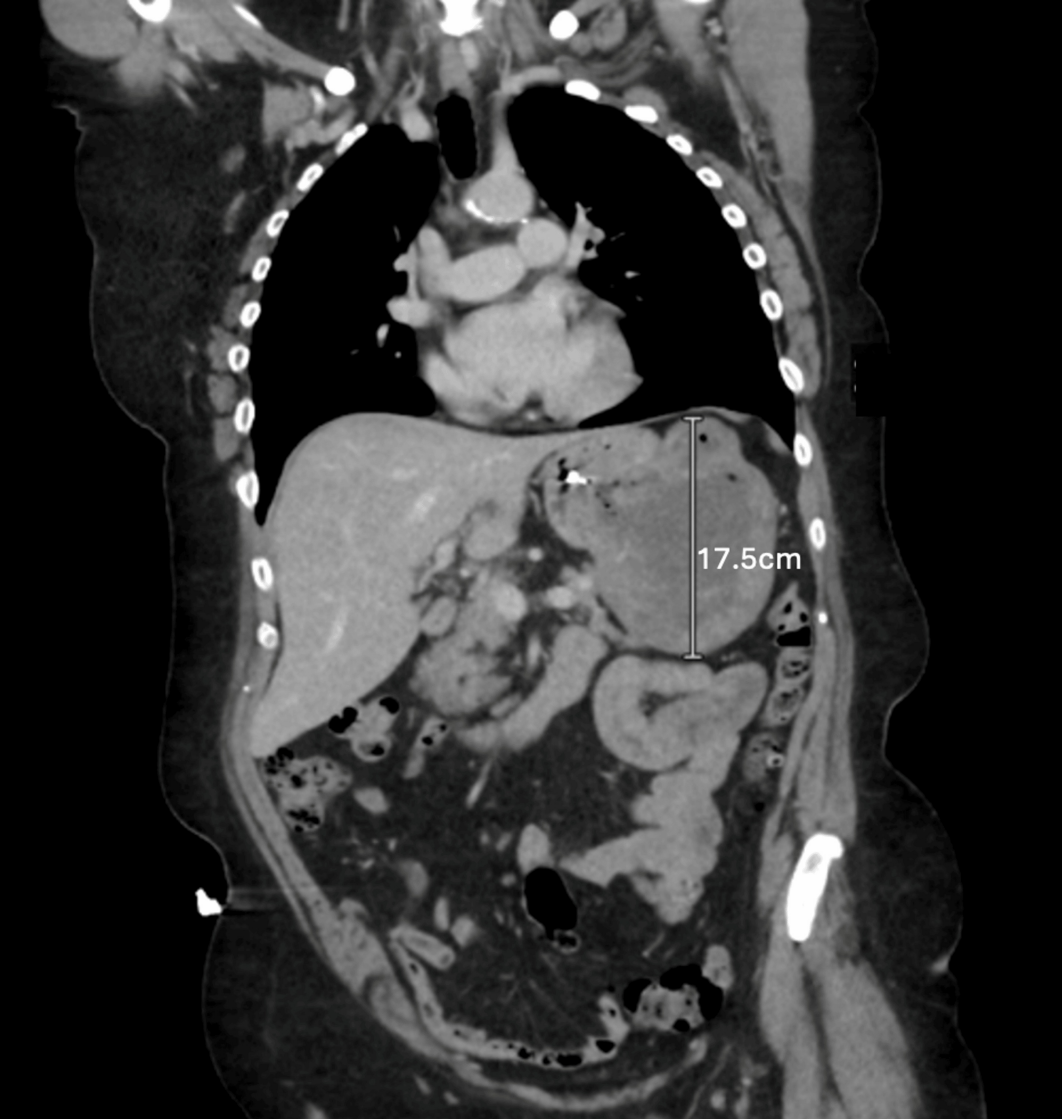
Abdominal CT scan with IV contrast visualizing a large exophytic gastric mass.
